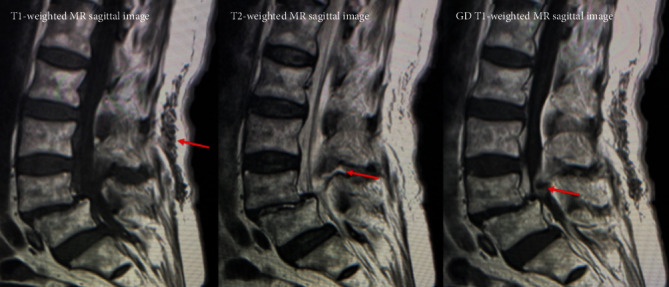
An intensity change of the L1-4 supraspinal ligament in T1-weighted images, effusion at the L3-4 interspinous space in T2-weighted images, and a GD-enhanced epidural mass at the level of L4 vertebral body.
